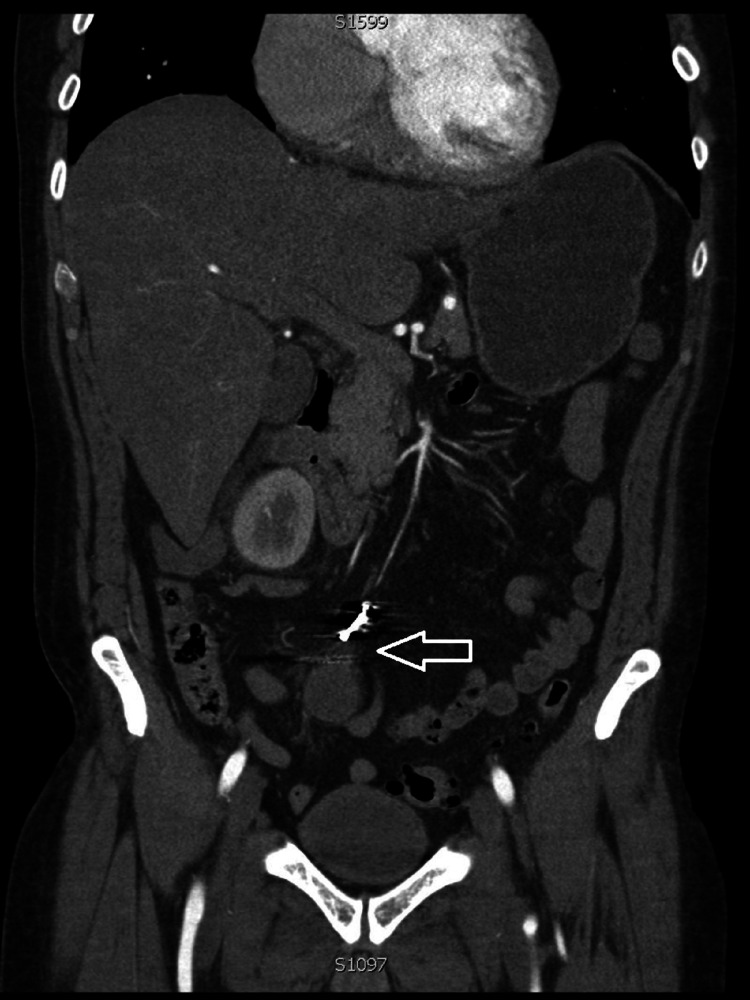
Coronal image of CT abdomen and pelvis revealed the reduced size of pseudoaneurysm with a coil in situ and no enhancement (arrow).
